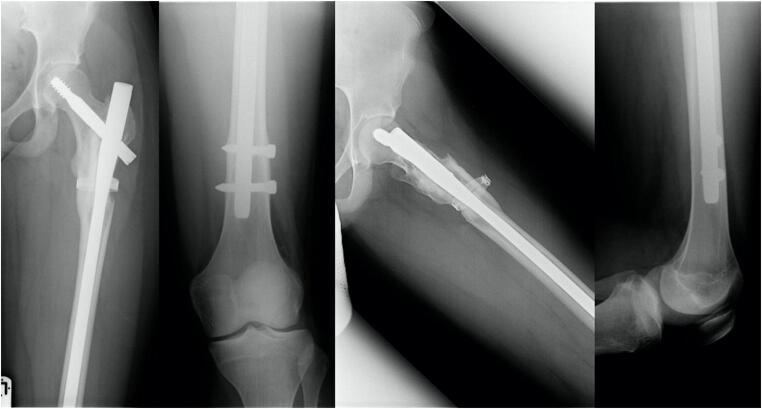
Anterior-posterior and axial X-ray of the left femur showing an atrophic pseudarthosis of the lateral cortex near the implantation site of the femoral neck screw (1 year postoperative)
